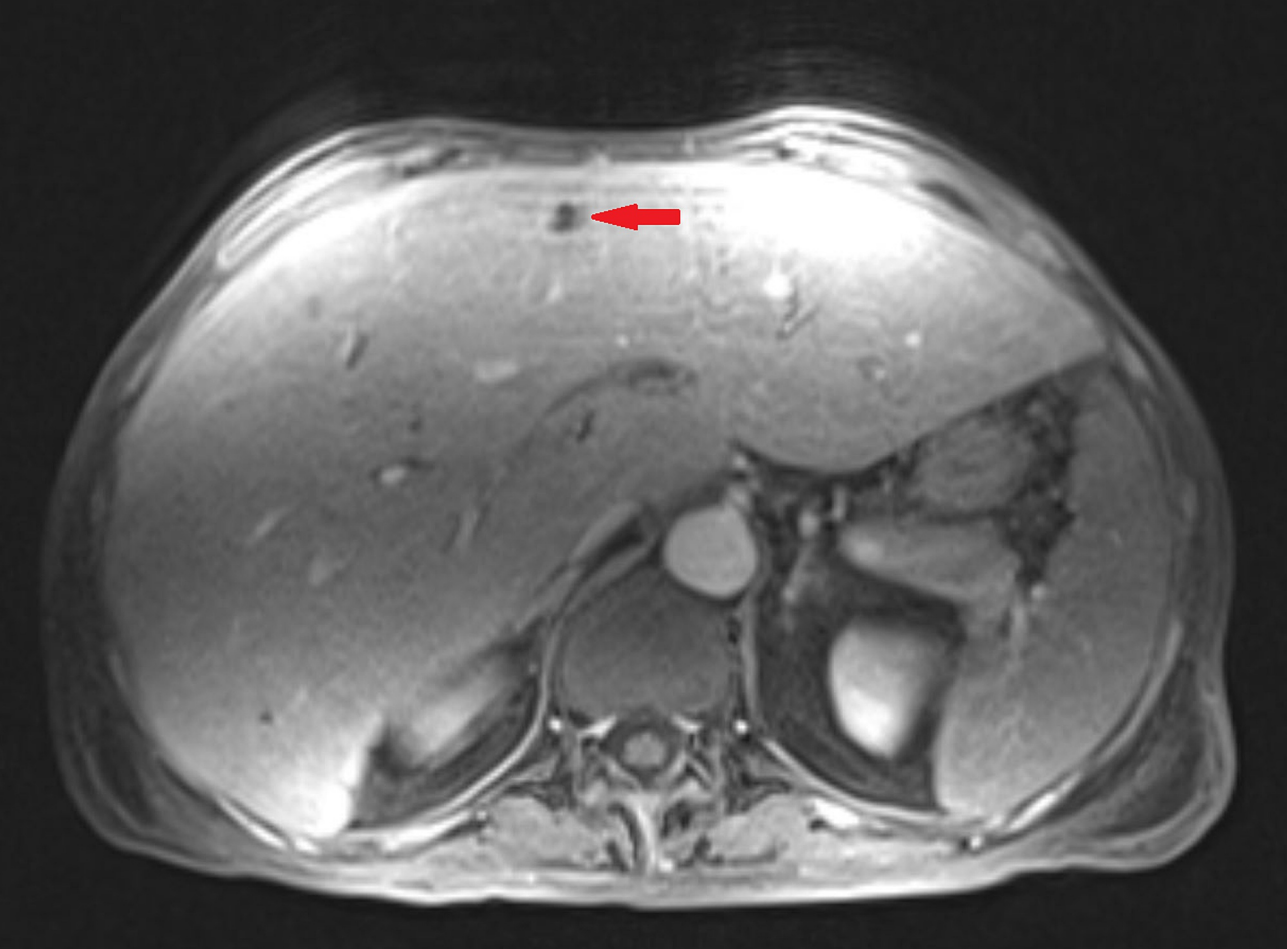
MRI of the liver showing a nodular hepatic lesion (red arrow).
